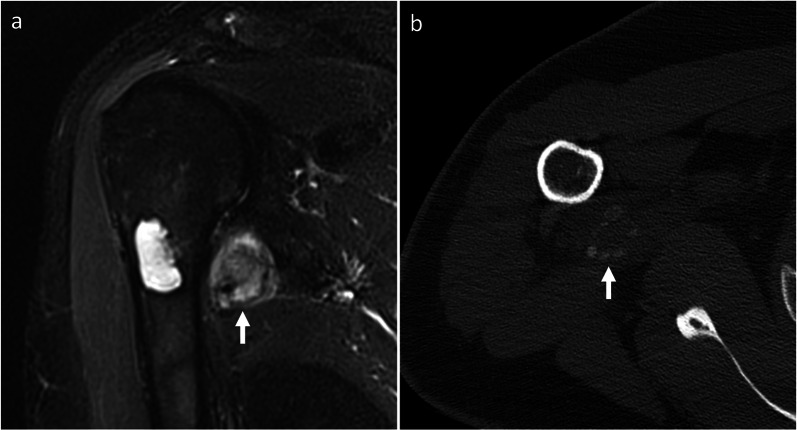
Myositis ossificans. On coronal fat-saturated T2-weighted MRI sequence (a), an intermediate-to-high signal mass is noted close to the medial aspect of the proximal humerus. This mass is separated from the underlying bone. A central chondroid lesion of the humerus is also seen. On CT (b), “zonal phenomenon” with peripheral mineralization and central lucency is shown. Myositis ossificans was pathologically proven. Arrow points at myositis ossificans in both images
